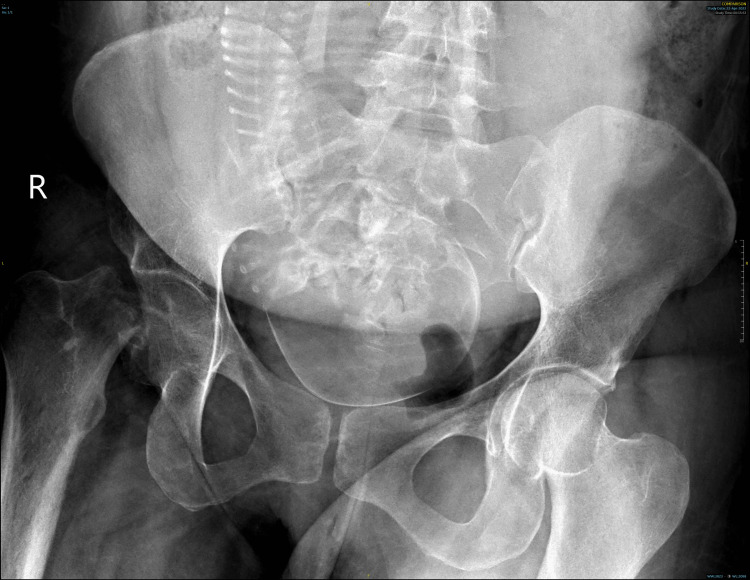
Pelvic X-ray post-trauma The X-ray shows a right femoral neck fracture with osteopenia of the right proximal femur and foetus in vertex presentation.
